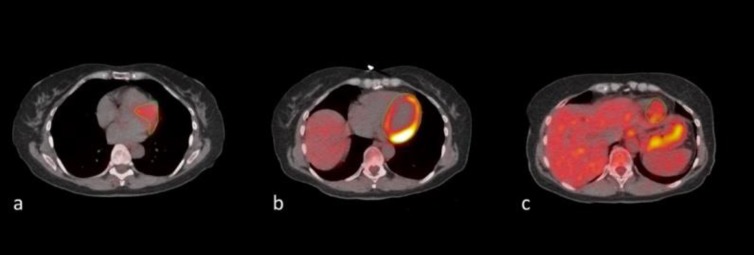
These scans belong to a 62 years old female. Regions of interest were manually drawn around the anatomical borders of the left ventricle on fused ^18^F-FDG PET/CT axial slices. (a=superior, b=middle, c= inferior)
